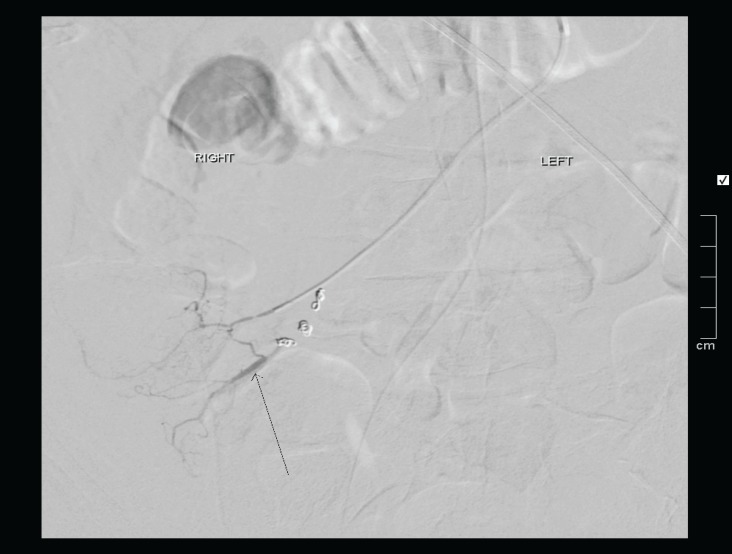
As the bleeding point was known to be from the caecum on CTA, a microcatheter was advanced into distal branches of the ileocolic artery and microcoils placed. After some coils were placed the branch demonstrating the bleeding point was identified (arrow
